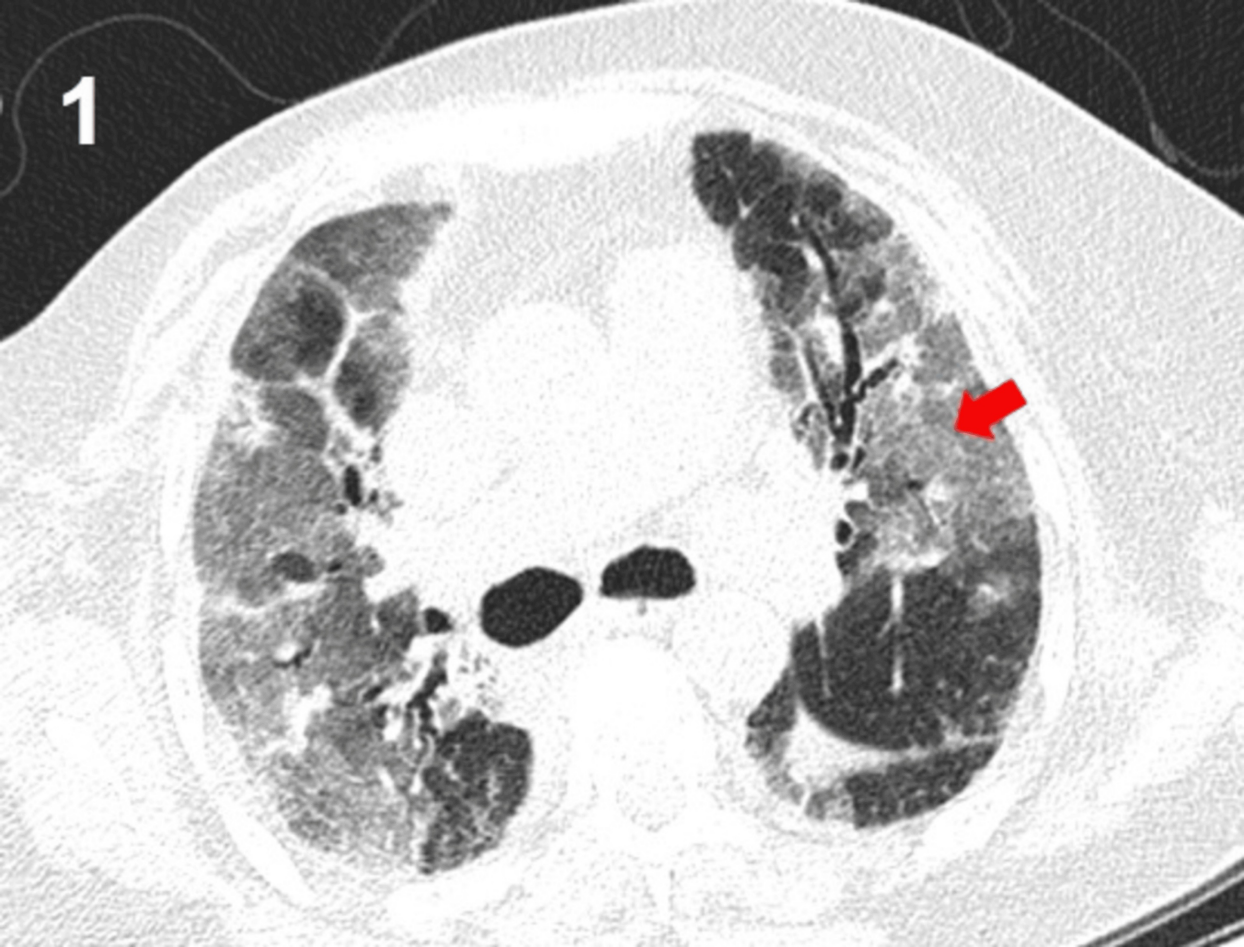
Computed tomography (CT) scan of the chest showing bilateral ground-glass opacities (red arrows) associated with intralobular and interlobular septal thickening, along with bilateral pleural effusions.
